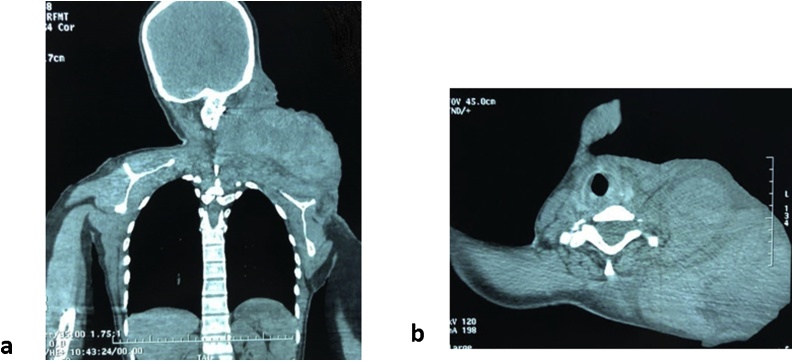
a: CT scan coronal section showing distal extension of the mass. b: Axial CT scan of the neck showing the mass with a permeable airway.
